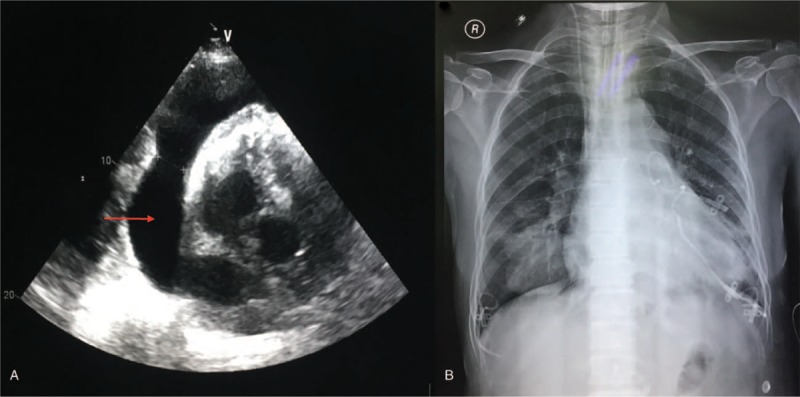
(A) Echocardiogram confirms the presence of a large pericardial effusion. (B) The chest X-ray reveals an increase in heart shadow.
